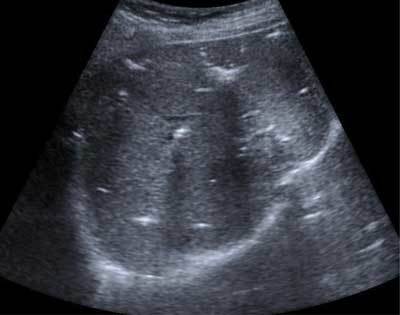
Ultrasound scan, axial view, of liver shows pneumobilia (black arrow).
